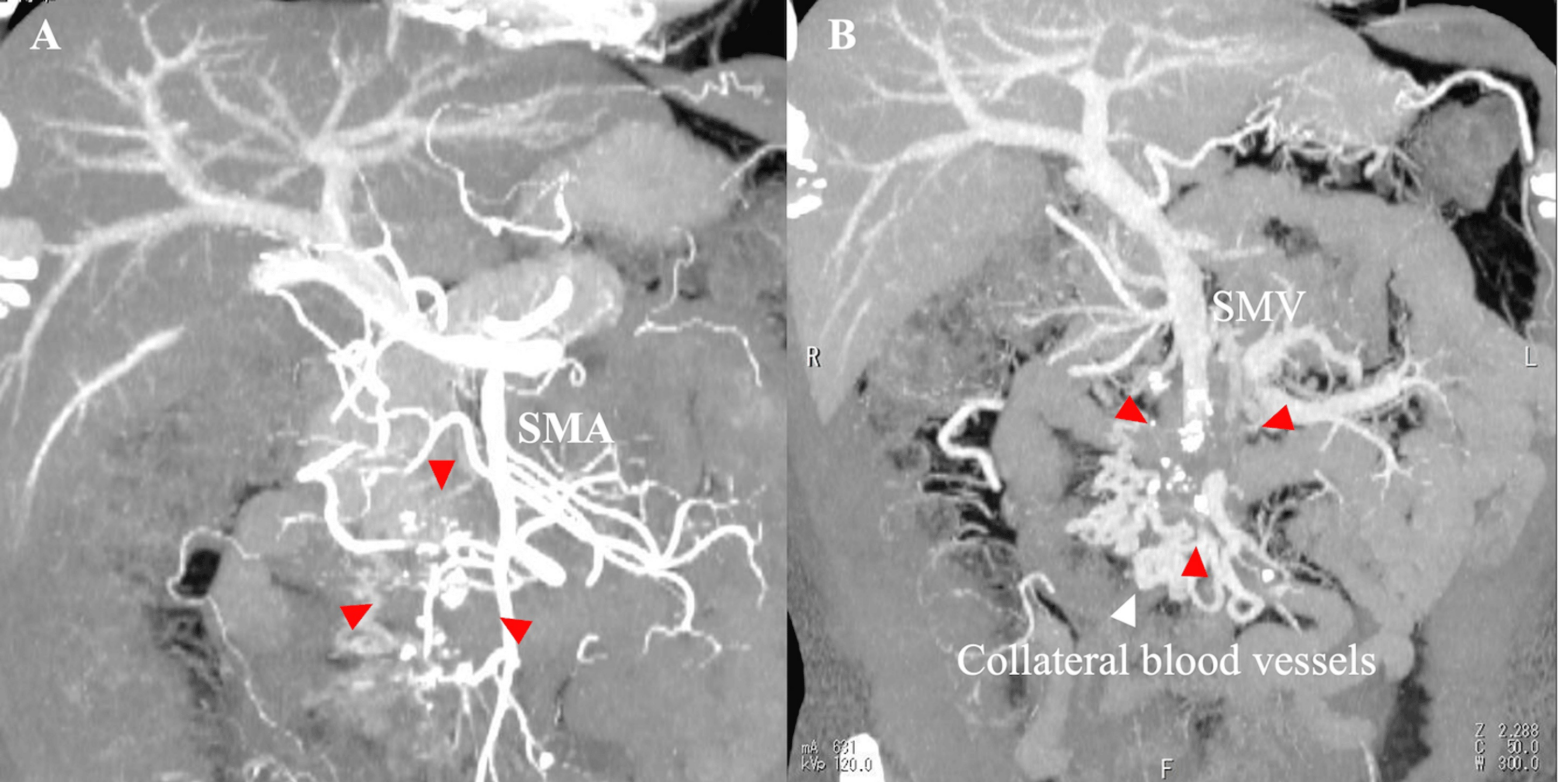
Computed tomography angiography (CTA). CTA revealed a mass, indicated by red triangles, in the arterial phase (A), invading the second and third branches of the superior mesenteric artery (SMA). The superior mesenteric vein (SMV) was occluded in the venous phase, with associated collateral vessels and dilated veins in the surrounding area, indicated by a white triangle.
